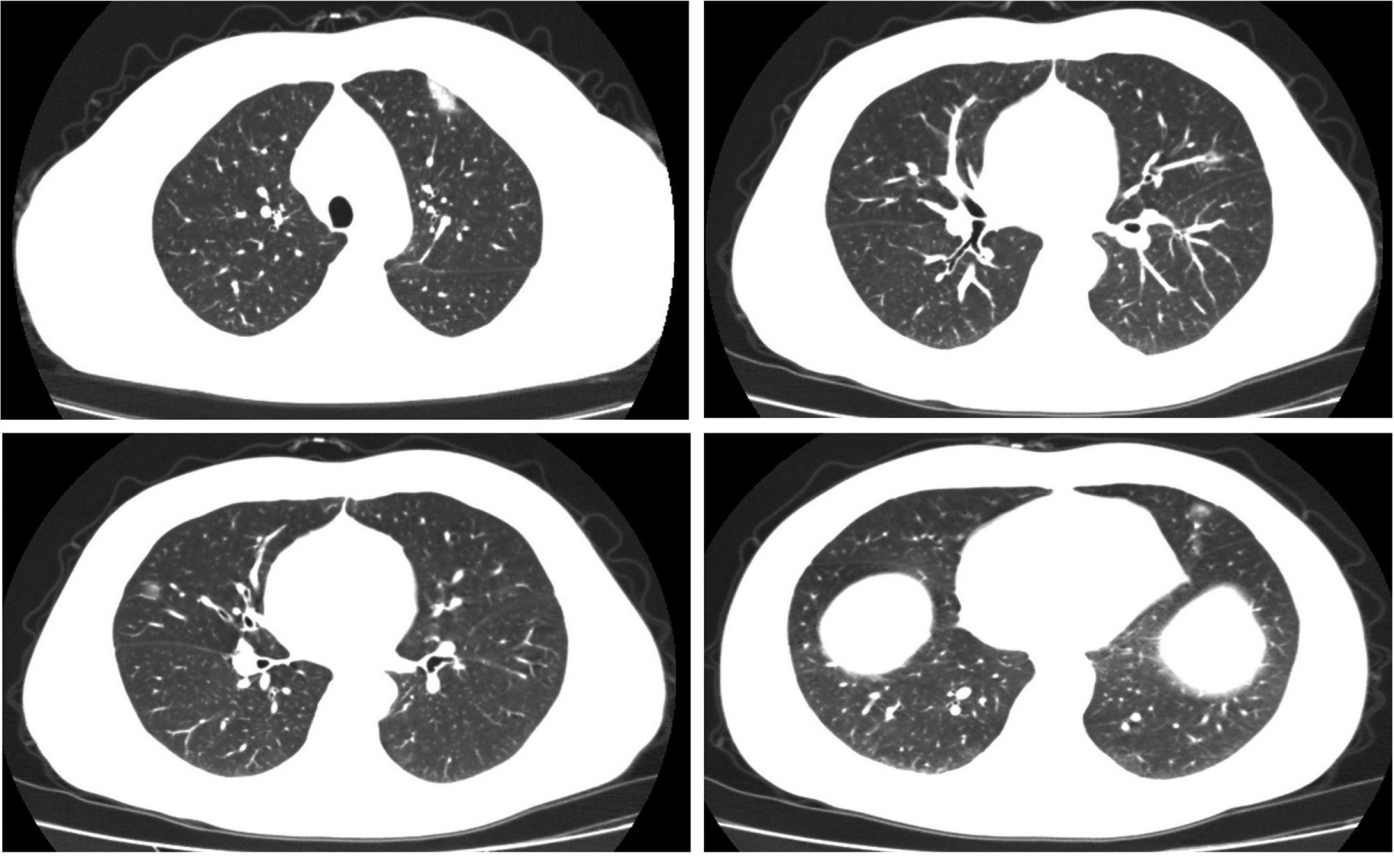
Case 2: CT chest result showed multiple peripheral ground-glass images on bilateral lungs.
